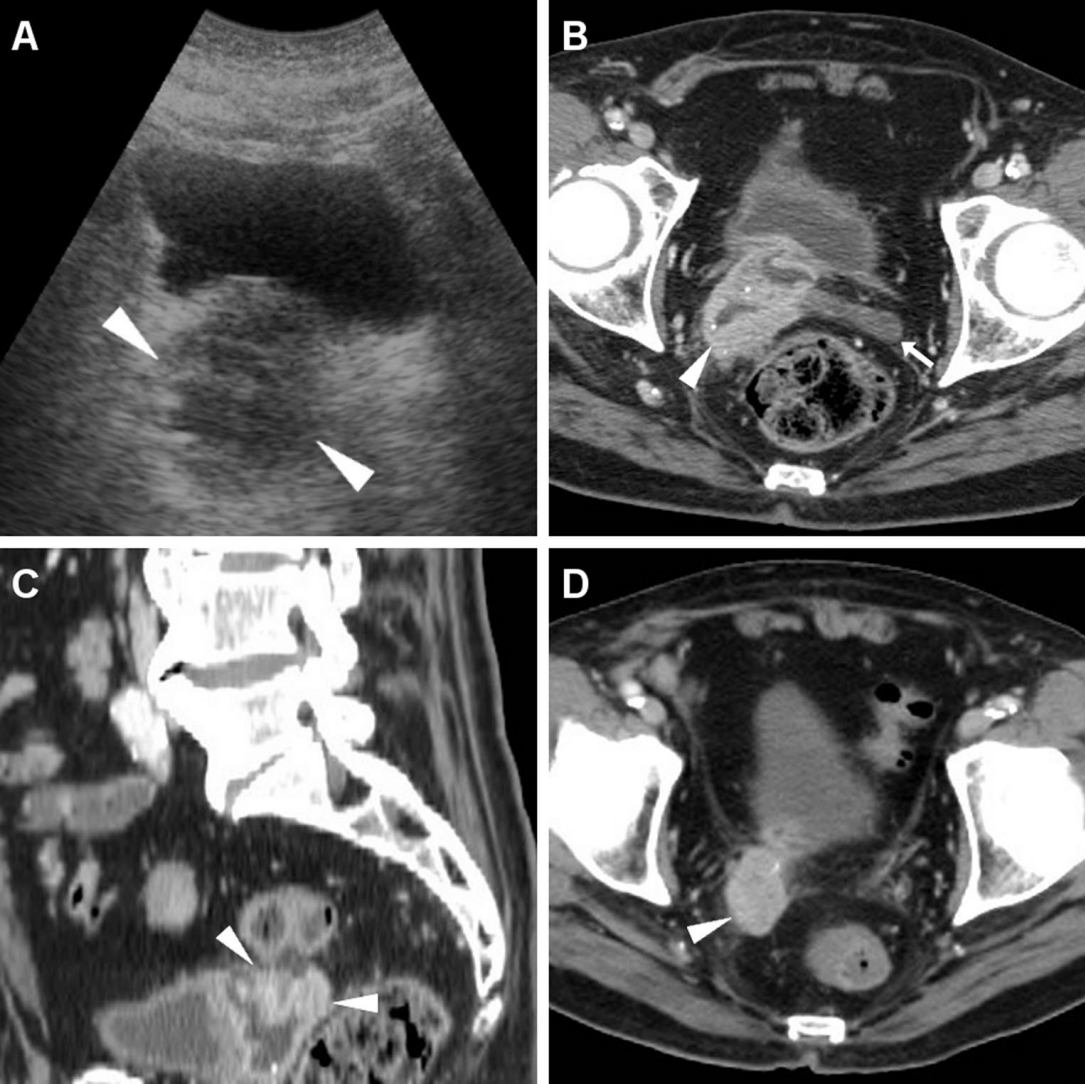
Seminal vesicle abscess in 74-year-old man with recurrent UTIs, suffering from malaise, persistent fever, pelvic tenderness and dysuria. Transabdominal ultrasound (a) revealed right paramedian inhomogeneous hypo-anechoic multiseptated mass (*arrowhead*), exerting compression on the urinary bladder. CT (b, c) confirmed markedly enlarged right seminal vesicle (*arrowheads*) with thick, strongly enhancing walls and septa, speckled calcifications, and internal liquefied areas. The abscess partially regressed, with disappearance of the mass effect and liquefied portions at follow-up CT (d), after intensive antibiotic treatment. Serum prostate-specific agent (PSA) normalised from 10 to 5 ng/mL over 2 months [Partially reproduced with permission from Ref. [54]]
